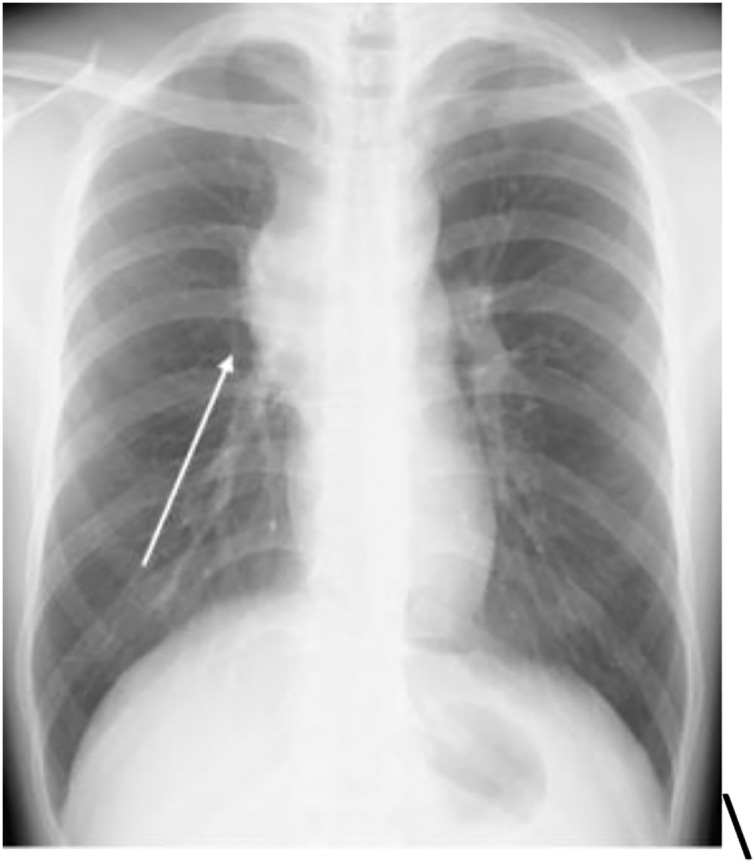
Chest radiograph (frontal view) showing a suspected tumor in the anterior mediastinum (arrow).
